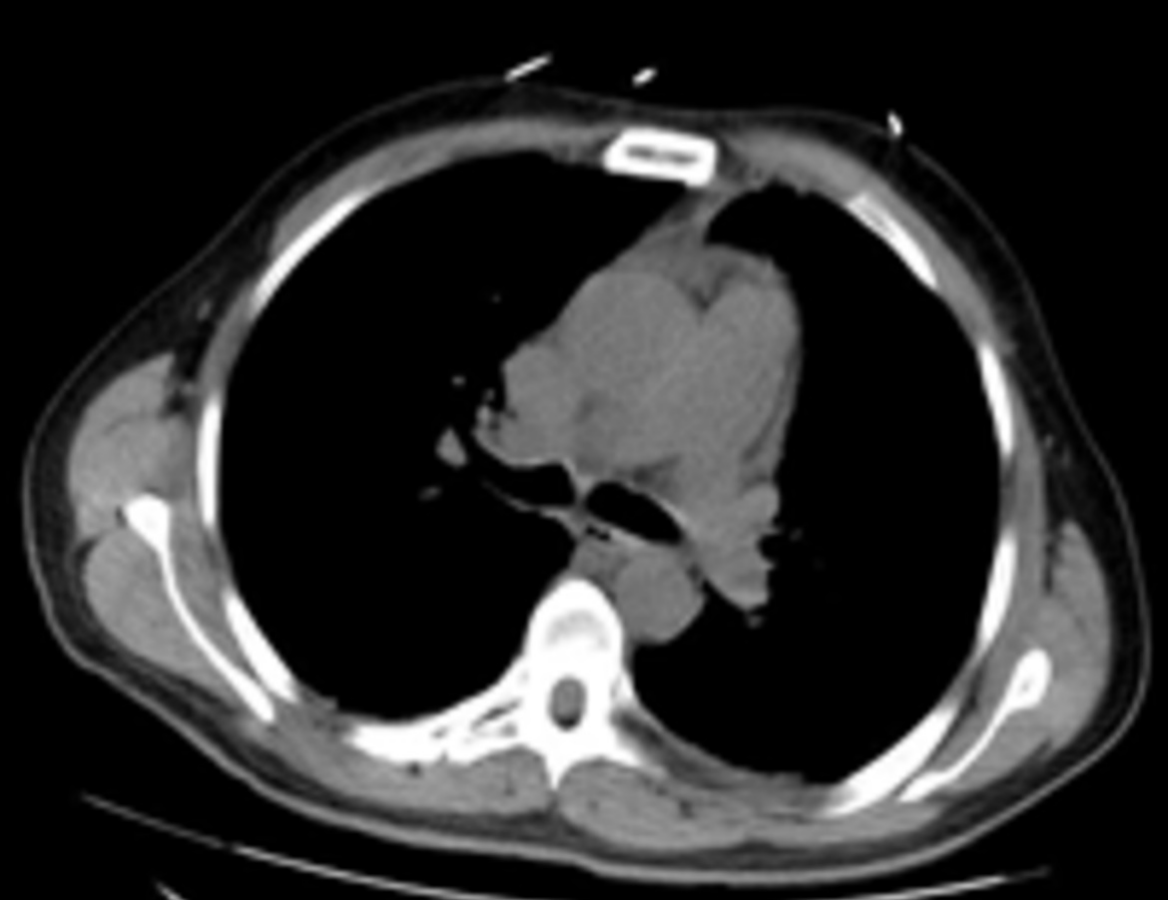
Computed tomography scan of the chest, six weeks prior to current presentation, showing no obvious lung lesion
